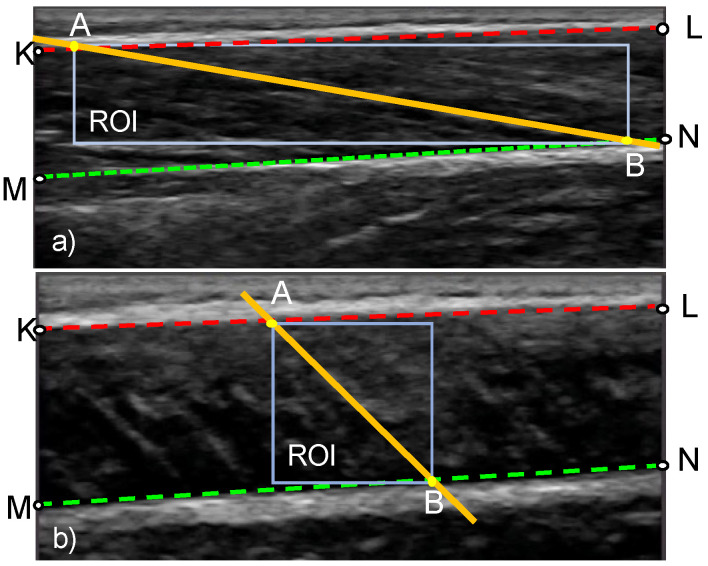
Definition of the region of interest (ROI) in an ultrasound image of a muscle at rest (a) and during maximal contraction (b). The geometric points that are used in the calculation of FL and PA are also shown. Lines KL and MN, respectively, correspond to the top and bottom boundary of the fascicle band. Points A and B denote the insertion points of the fascicle direction line.
